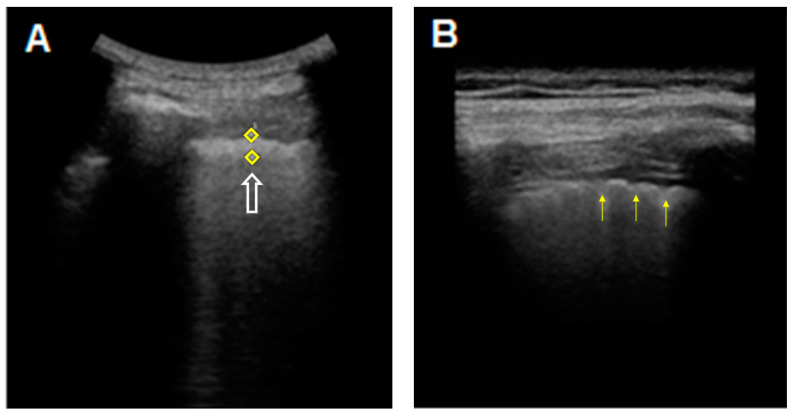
TUS images of the hyperechoic pleural line in a patient with an HRCT pattern of Usual Interstitial Pneumonia (UIP). (A) The hyperechoic pleural line (white arrow) appears thickened (5.1 mm) when measured with a middle/low-frequency convex probe (3.5–5 MHz). (B) The thickened (2.4 mm), irregular, fragmented and blurred appearance (yellow arrows) becomes more evident when using a high-frequency linear probe (8–12.5 Mhz).
